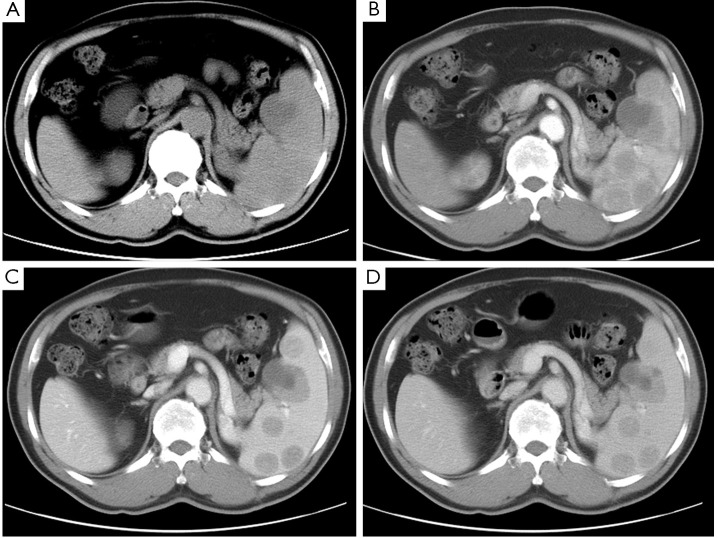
Abdominal computed tomography images of the patient. A. Plain scan; B. Contrast-enhanced scan at the arterial phase; C. Contrast-enhanced scan at the portal venous phase; D. Contrast-enhanced scan at the delayed phase.
